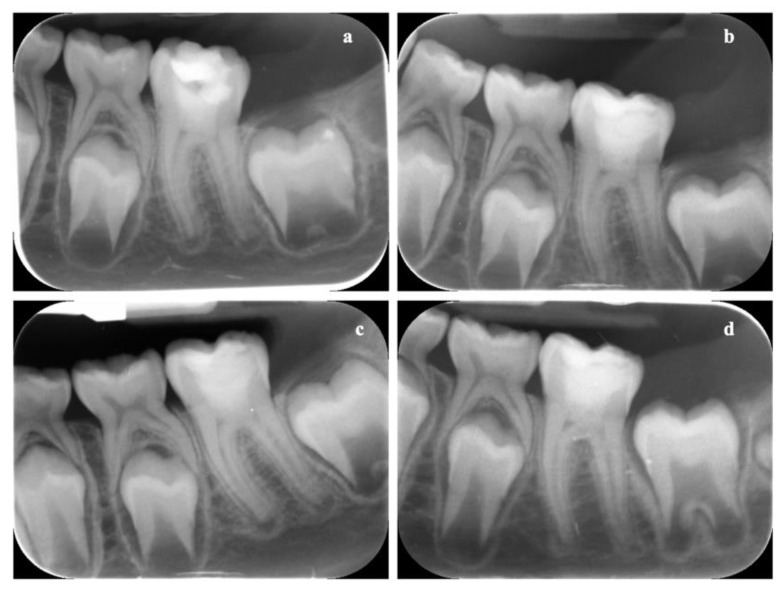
Periapical radiographs of the dental element (3.6) treated with Biodentine. The immature permanent tooth is shown immediately after vital pulp therapy (Baseline) (a) and after 1 month (b), 3 months (c), and 1 year of follow-up (d). Progressive formation of the dental roots could be observed over time with an absence of periapical reaction.
